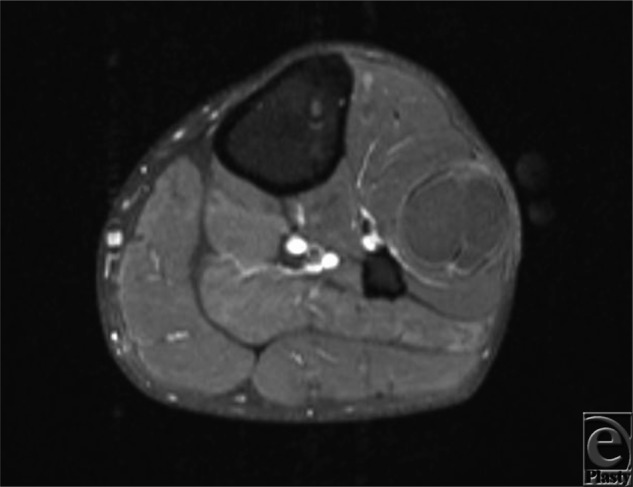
Axial contrast-enhanced, T1-weighted magnetic resonance image of the peroneus longus ganglion cyst, well-circumscribed.
